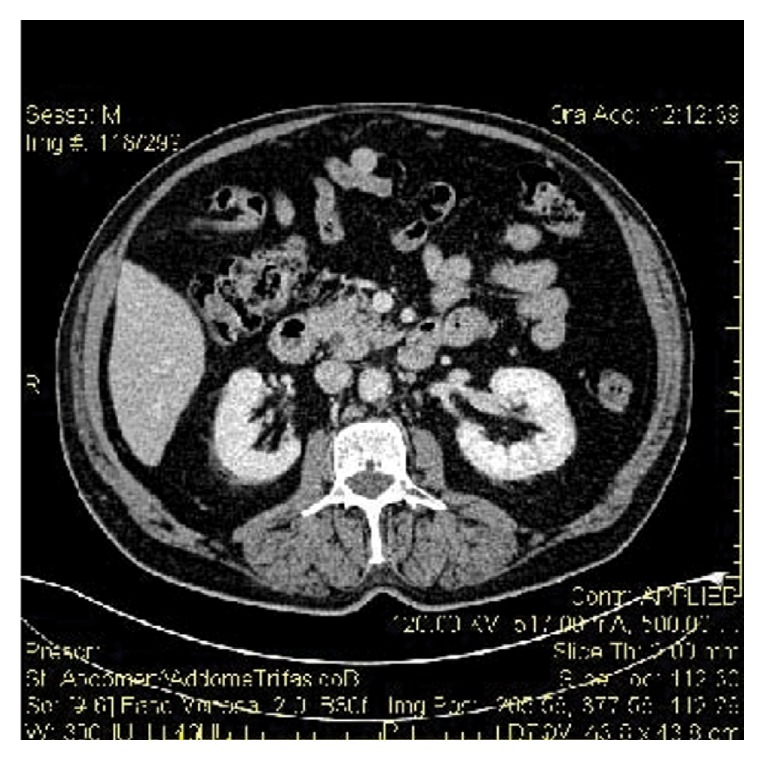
Follow-up CT venous phase.
